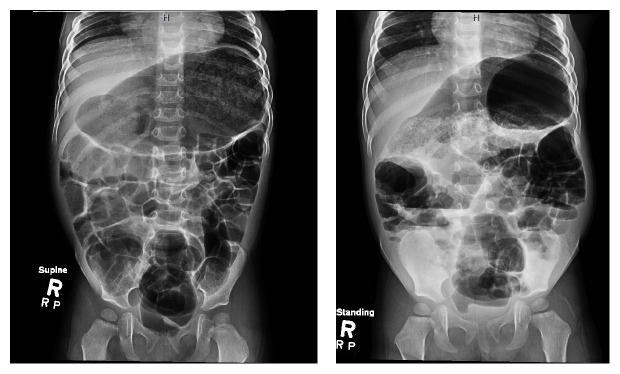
Supine and upright plain X-rays of the patient presented in Case 1. Moderate dilatation of the bowel is seen with significant gaseous distention of the stomach being seen. Multiple air-fluid levels are seen. No free intra-abdominal air is identified.
